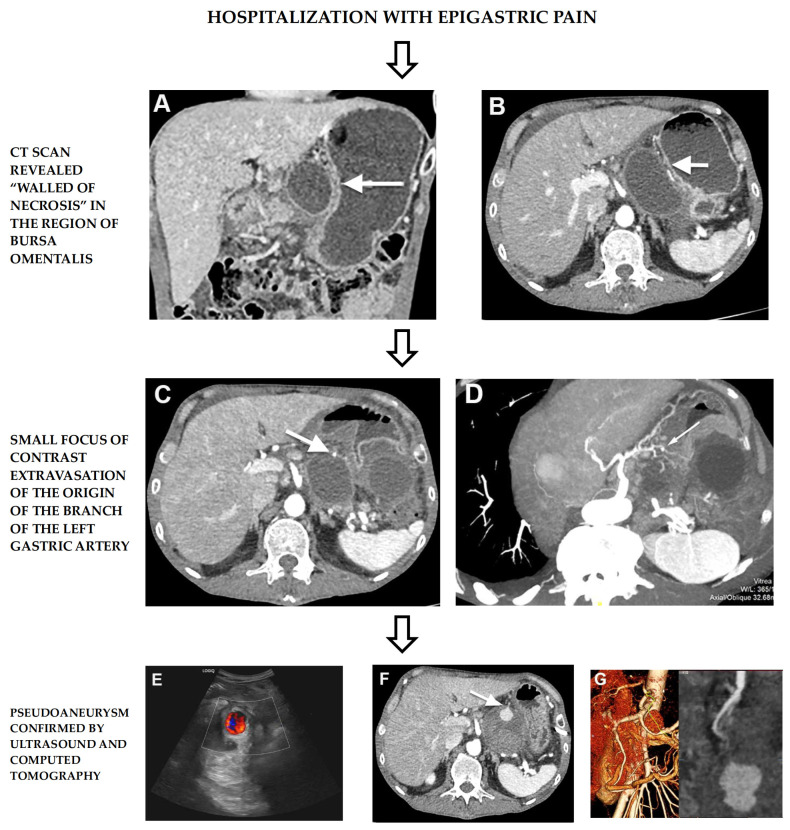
Well-defined cystic mass in the region of bursa omentalis—WON (A,B). Small focus of contrast extravasation suspected on pseudoaneurysm formation (C,D). Yin-yang sign on abdominal ultrasound with CD corresponding to turbulent blood flow in formed pseudoaneurysm (E). CT presentation of pseudoaneurysm originating from left gastric artery within WON (F,G).
